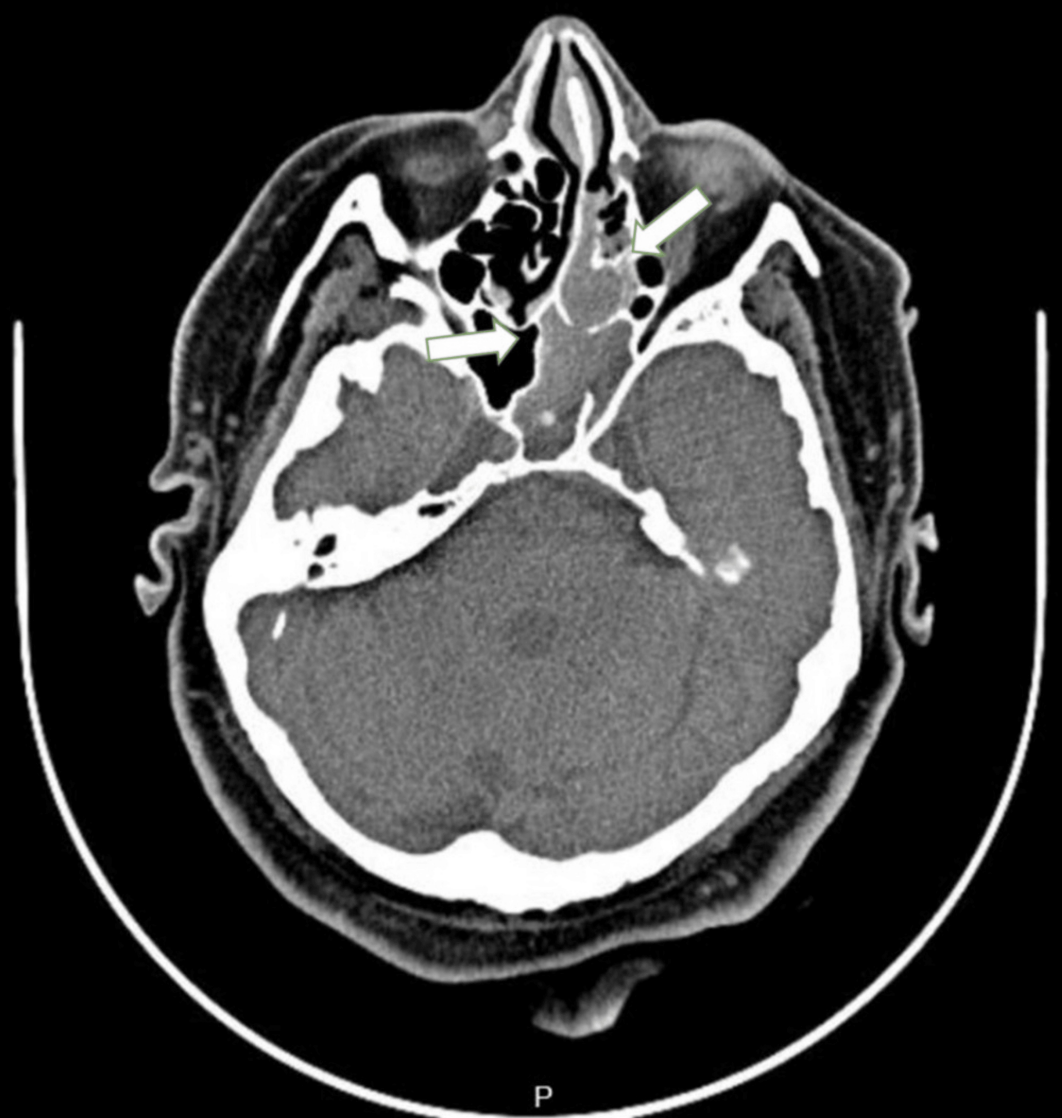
Plain CT scan showing an isodense mass in the right ethmoid sinus extending to the sphenoid sinus. CT: computed tomography
